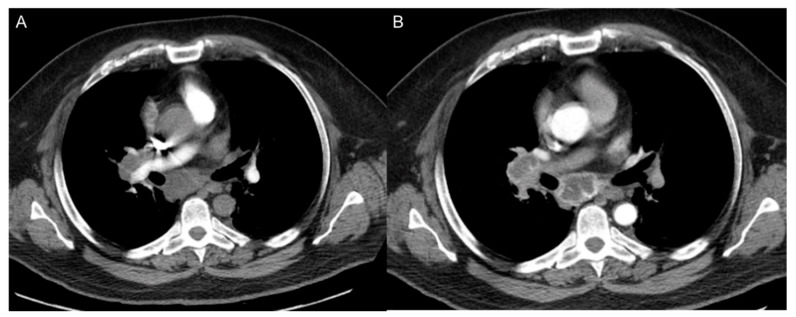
CT perfusion of a right hilar lesion was performed following administration of IV contrast. Displayed are selected sequential acquisitions (A,B) collected for measurement of perfusion parameters.
